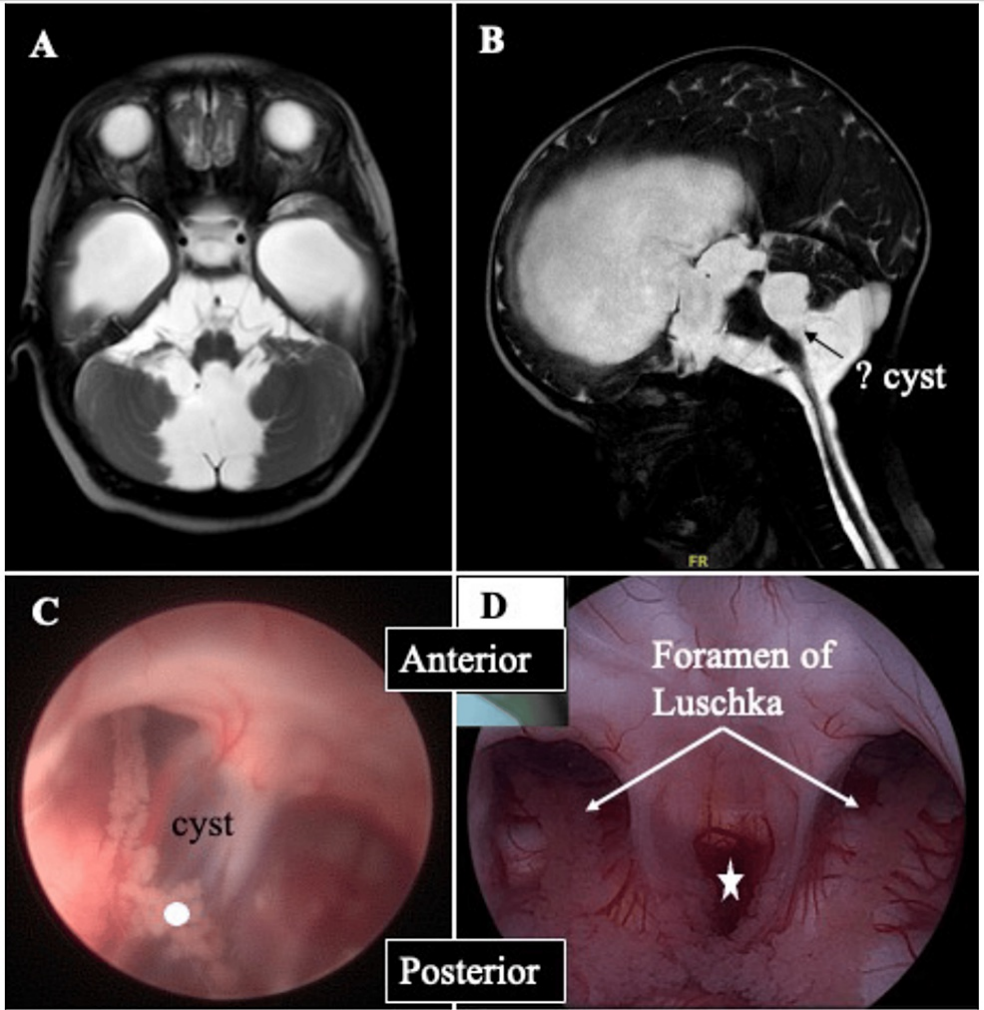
A 14-year-old boy presented with raised intracranial pressure. (A, B) The axial and sagittal T2-weighted brain MRI shows the cystic dilatation of the fourth ventricle, filling the posterior fossa. The image also showed a layer of arachnoid membrane at the lower level of the fourth ventricle. The patient underwent an endoscopic guided fenestration of the cyst. (C) The arachnoid membrane is visualized, and posterior to it is the choroid plexus of the fourth ventricle (circle). (D) Following endoscopic fenestration, CSF patency is established, and the foramen of Magendie (star; midline) and the foramen of Luschka (lateral bilaterally) are visualized.
